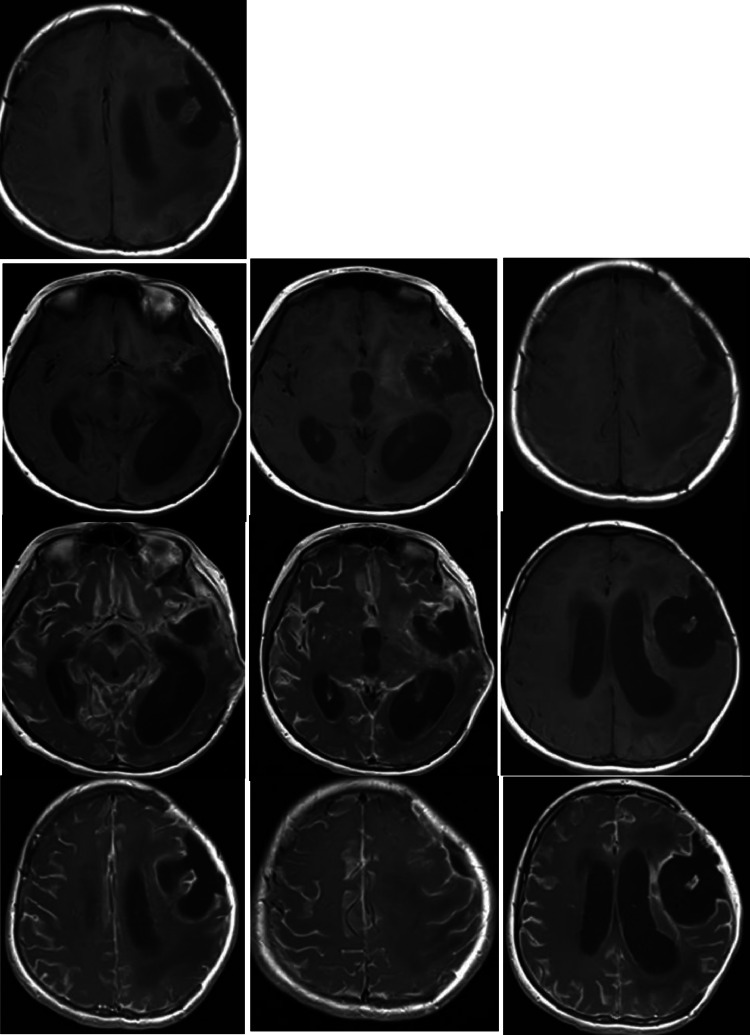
Post-contrast MRI brain: Heterogeneously enhancing left frontoparietal mass. The postcontrast T1-weighted MRI of the brain reveals an excisional cavity with marginal enhancement. There is also diffuse hydrocephalus.
